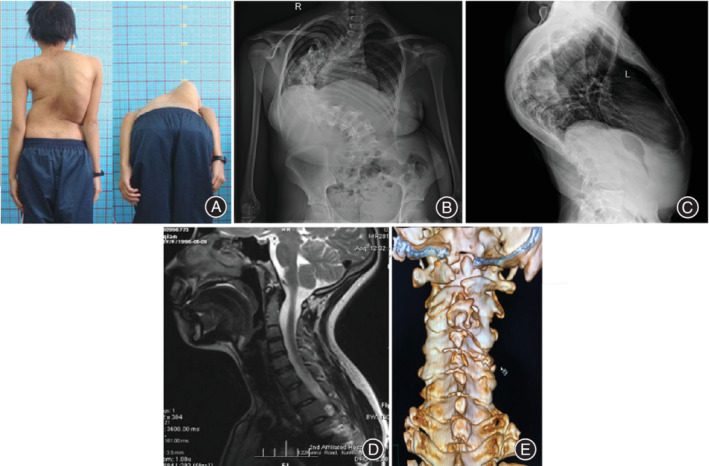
A 20‐year‐old female with severe kyphoscoliosis associated with cervical osseous abnormalities (COA). The preoperative appearance reveals spinal deformity (A). The preoperative AP and lateral radiographs show the severe kyphoscoliosis (B, C). The MRI and CT scanning of the cervical spine (D, E) show a variety of COA manifested in combination.
